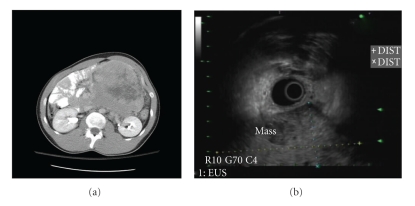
(a) CT film showing large mass displacing small bowel to the right. The mass measured 12.8 × 13 × 12.5 cm arising from the retroperitoneum and was displacing the 3rd and 4th portions of the duodenum to the right. (b) EUS image of large hypoechoic mass adjacent to the gastric wall, measuring 9.6 × 7.4 cm. The distal aspect of the mass was beyond the reach of the ultrasound probe.
